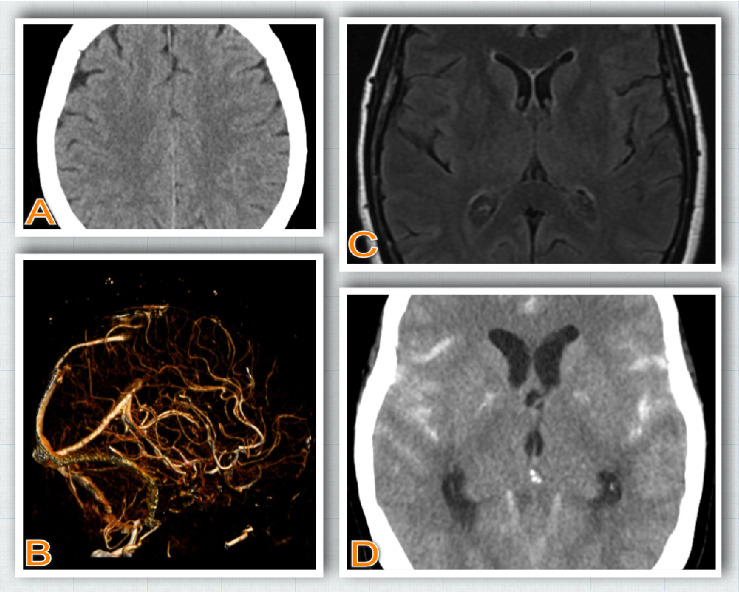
(A) Day 1 CT scan (coronal slice): no hemorrhage. (B) Day 1 CT angiogram (3D reconstruction): no evidence of aneurysm. (C) Day 2 MRI brain: normal. (D) Day 2 CT Head: Diffuse subarachnoid hemorrhage.
